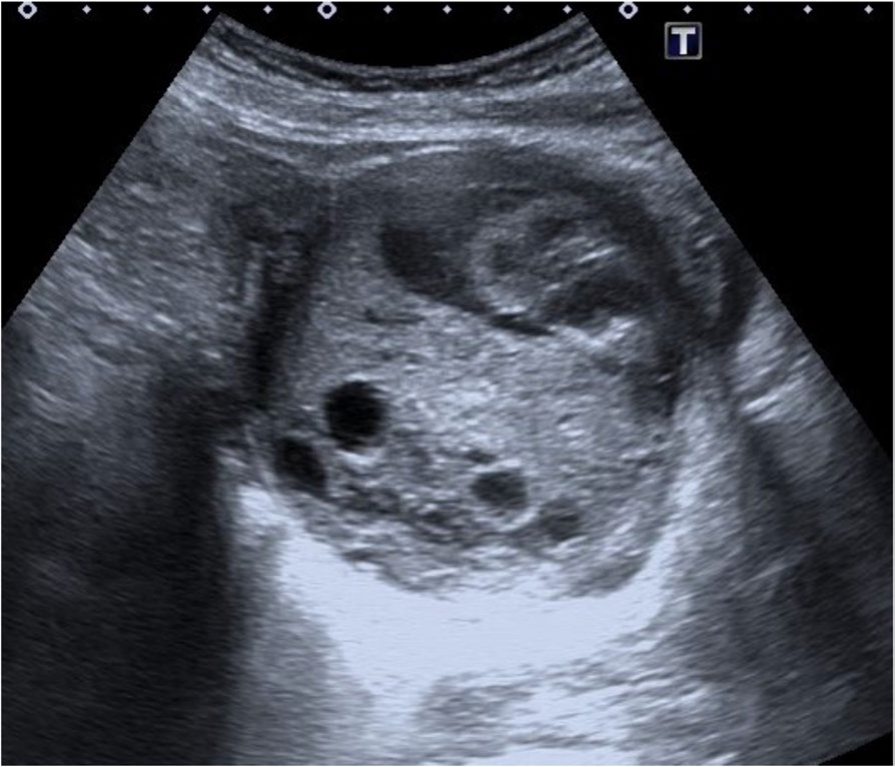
Transabdominal ultrasound. Large pelvic mass located below the corpus uteri. The lesion is heterogeneous with echogenic and anechoic areas, showing good US through-transmission which resembles fibroids with hyaline or cystic degeneration. Some Doppler signal was detected on the echogenic parts of the mass (not shown)
